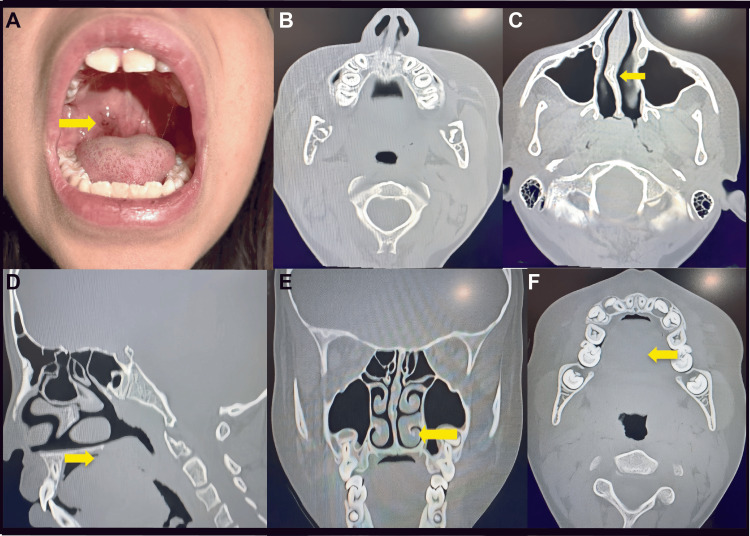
Representative images from the physical examination of our patient and the CT scan of the nose and paranasal sinuses. A. Well-defined, cystic-appearing lesion with fluctuating size during upper respiratory tract infections (URTIs) and their remission (yellow arrow). B. Axial section at the level of the palate that does not show the lesion. C. Axial section showing septal deformity in Cottle's area II to IV block with a contacting spur (yellow arrow). D. Sagittal section that does not show the lesion in the palate. E. Coronal section showing turbinate hypertrophy (yellow arrow). F. Axial section with no evidence of a cystic lesion.
